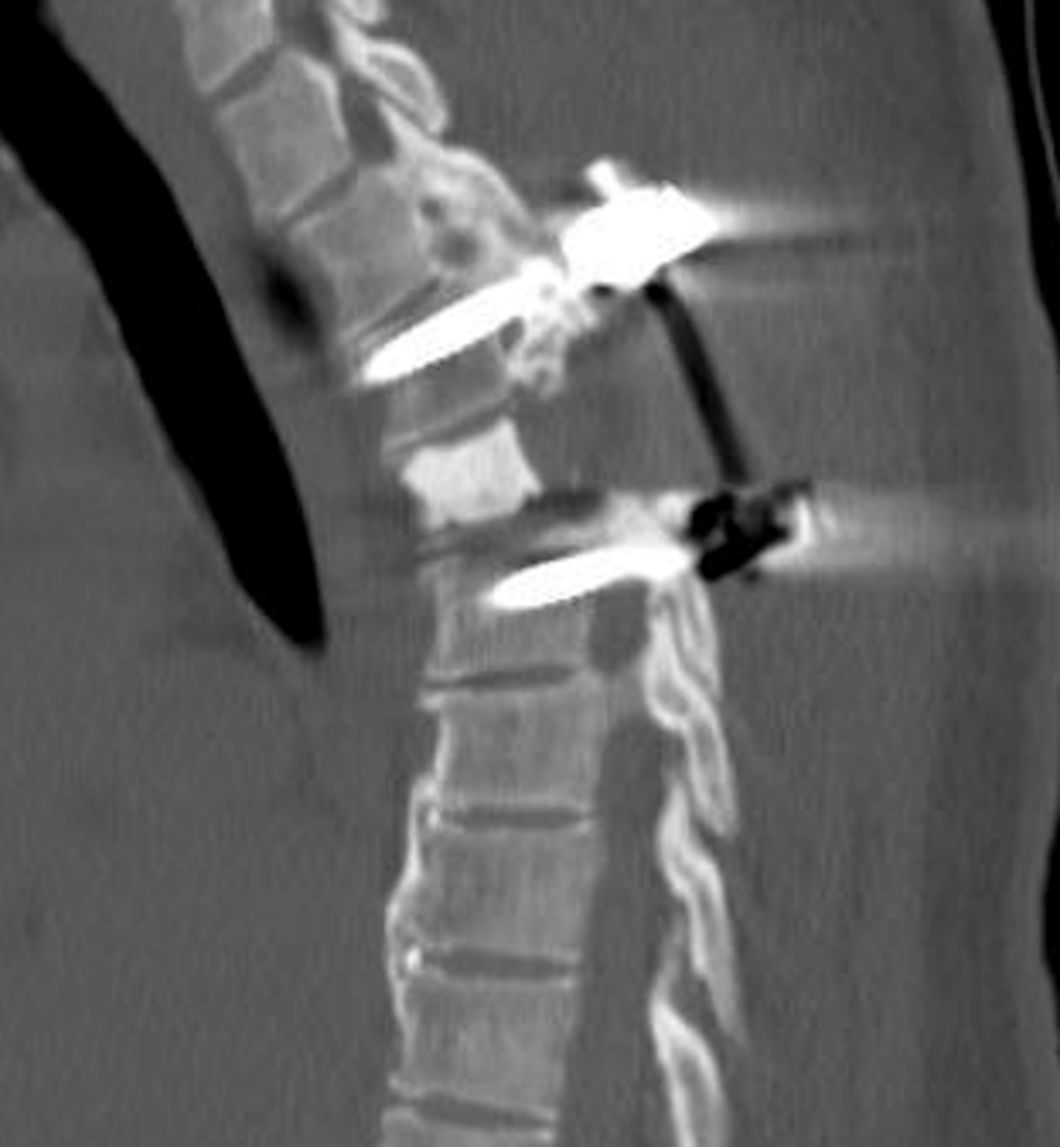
Postoperative CT scan depicting instrumented fusion with anterior PMMA support CT (Computed Tomography), PMMA (Poly-methyl methacralate)
